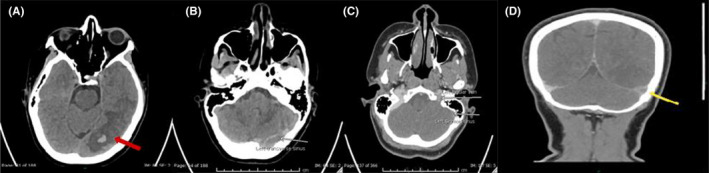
(A‐D) Plain‐ none enhanced CT head and CTV. (A, B) Left temporo‐occipital intraparenchymal hemorrhage with surrounding edema and adjacent mass effect evident by effacement of the adjacent cortical sulci. A cord like hypodensity noted in the left transverse sinus [white arrow]. (C, D) Filling defect involving the left transverse (yellow arrow) and left sigmoid sinuses extending to the left jugular vein. Left vein of Labbe is also not visualized [white arrows]
